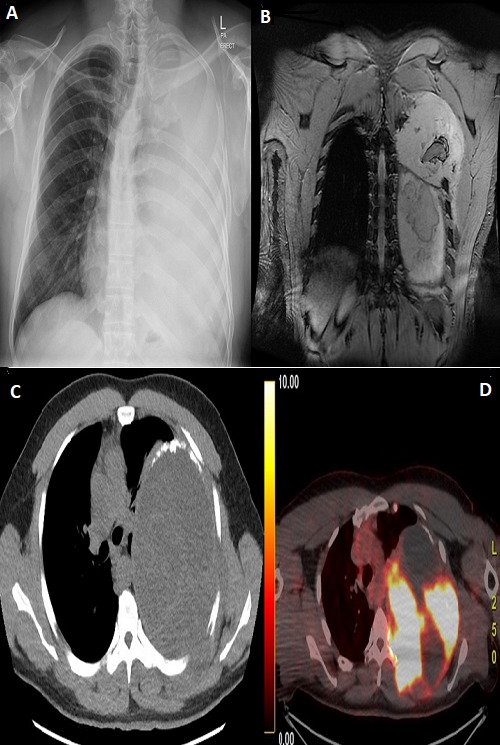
A) left sided chest opacity; B) MRI scan showing total collapse of left lung due to solid homogenous cystic mass invading 3rd, 4th, 5th, 6th ribs with the mass bulging posteriorly infiltrating the left para-spinal musculature; C) CT showing similar findings described on MRI scan in addition to the mass containing solid and cystic parts being inseparable from the chest wall; D) PET CT scan showing hyper metabolic mass involving the left hemithorax
